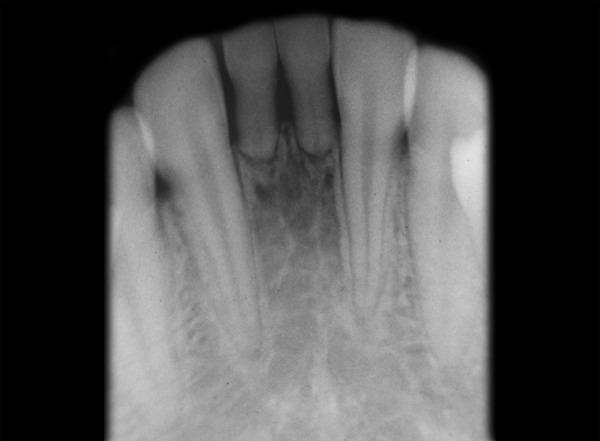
Periapical radiograph showing agenesis of both mandibular central incisors
